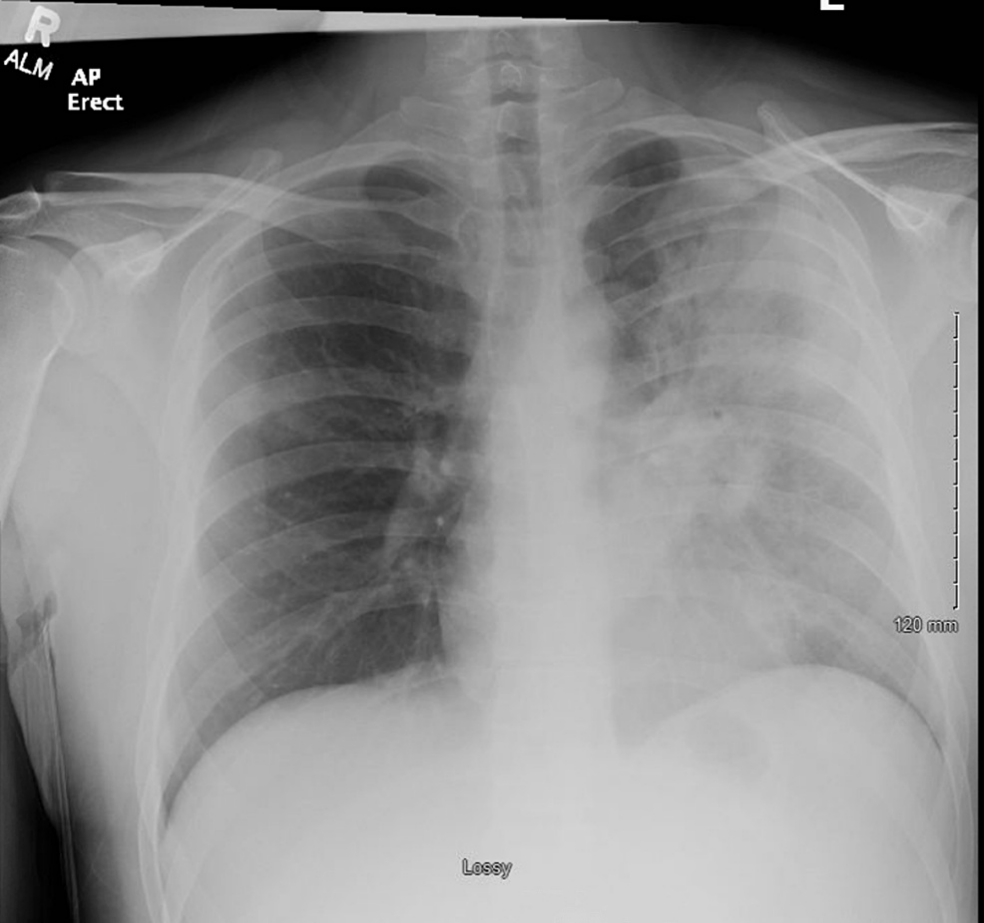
Chest x-ray demonstrating multilobar pneumonia
